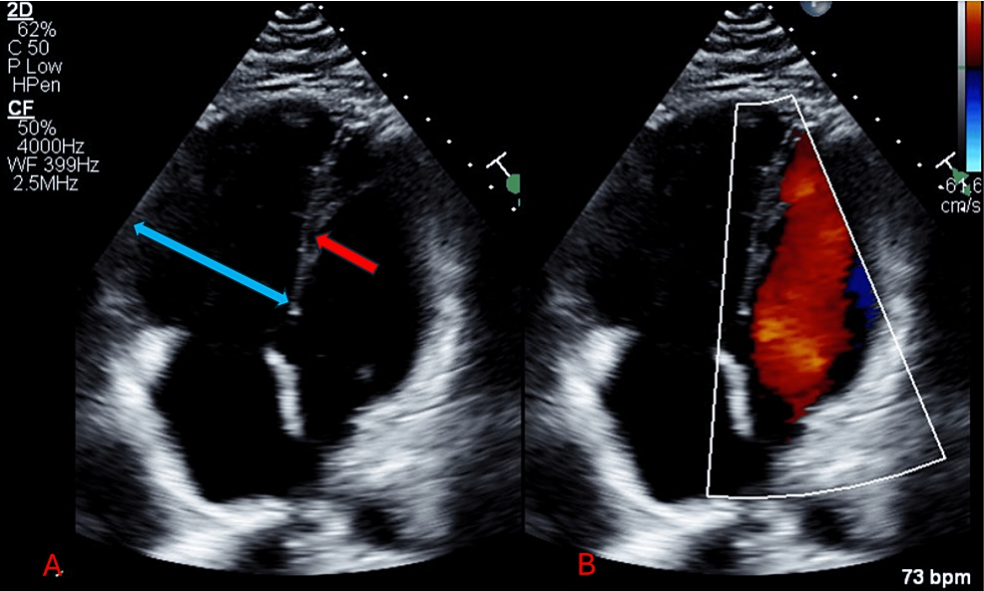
A) Echo showing a dilated RV (blue arrow) indicative of right heart strain and a flattened interventricular septum (red arrow); B) The left ventricle displays a normal configuration with a flattened interventricular septum (from last admission in 2024) Echo: Echocardiogram; RV: Right ventricle
